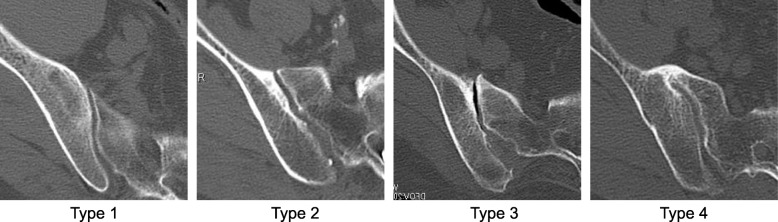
Variation of sacroiliac joint changes on computed tomography. Type 1, normal or tiny peripheral bone irregularity; Type 2, subchondral bone sclerosis and osteophytes formation; Type 3, vacuum phenomenon of SI joint; Type 4, bridging osteophyte and bony fusion of SI joint
